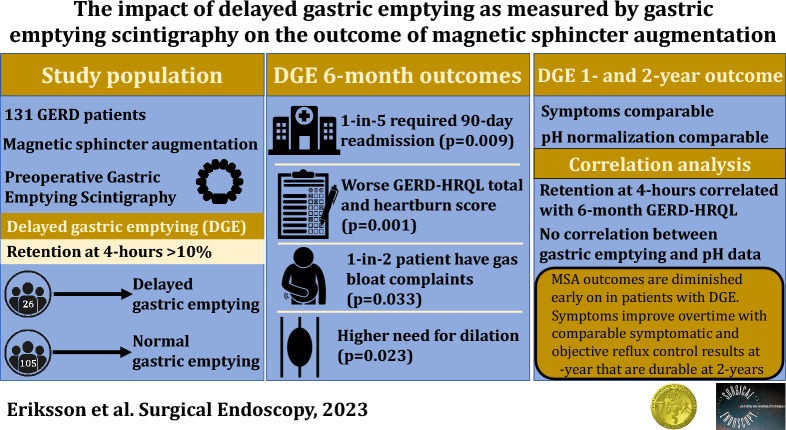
Delayed gastric emptying (DGE) is a defining characteristic of gastroparesis and a common finding in patients with gastroesophageal reflux disease (GERD). In fact, one-in-ten patients referred for antireflux surgery are found to have DGE on gastric emptying scintigraphy (GES) [1, 2]. However, the impact of objective DGE on ARS is controversial. Several studies have demonstrated higher recurrence rates among patients with DGE after fundoplication [2–4]. However, some studies have demonstrated no detrimental impact on ARS outcomes [5].
